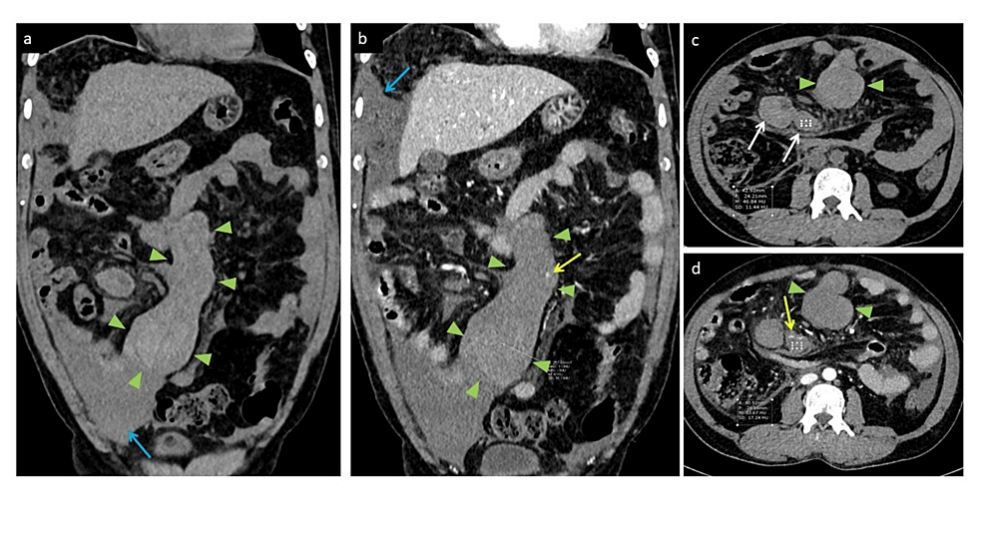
Non-contrasted (a, c) and contrasted (b, d) abdominal computed tomography A large lobulated mesenteric structure (arrowheads) involving the small bowel mesentery extending from the mesenteric root towards the right iliac fossa and measures 15 cm at maximal dimension. This structure shows high density in the non-contrast study and no post-contrast enhancement with surrounding fat stranding representing a mesenteric hematoma. This hematoma appears to displace the surrounding small bowels with no evidence of bowel wall thickening, decreased enhancement or intestinal obstruction. Other two smaller rounded adjacent similar hematomas (white arrows) are noted to the right side. Tiny contrast blushes were noted within these hematomas (yellow arrows) which suggest possible contrast extravasation. Mild amount of free fluid in the upper and lower abdomen (white arrows).
